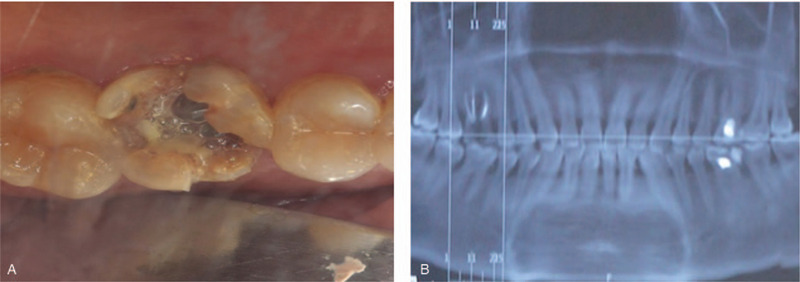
Preoperative and intraoperative photographs of case 1. (A) Clinical view of the tooth 16; (B) Radiographic aspect of the tooth.
